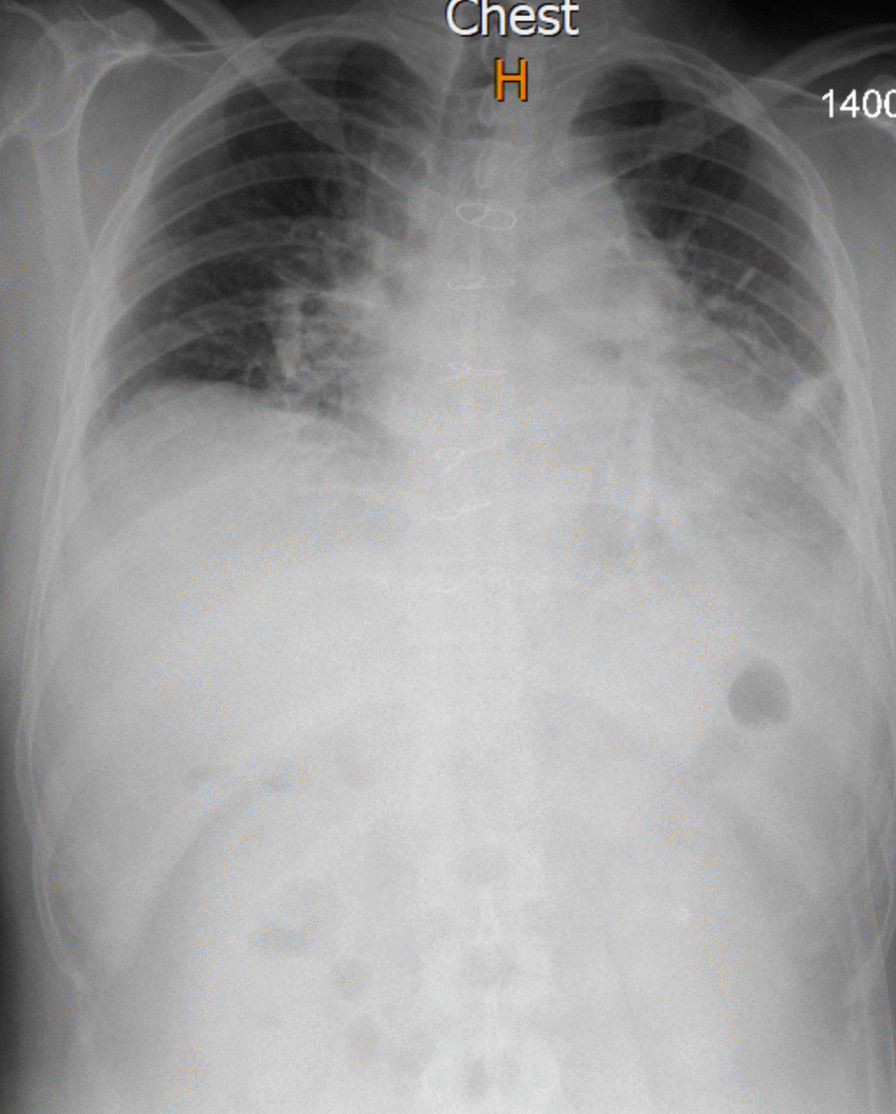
Post-operative chest X-ray of 48-year-old male patient undergoing Cavernous Hemangioma of mediastinum tumoral resection
